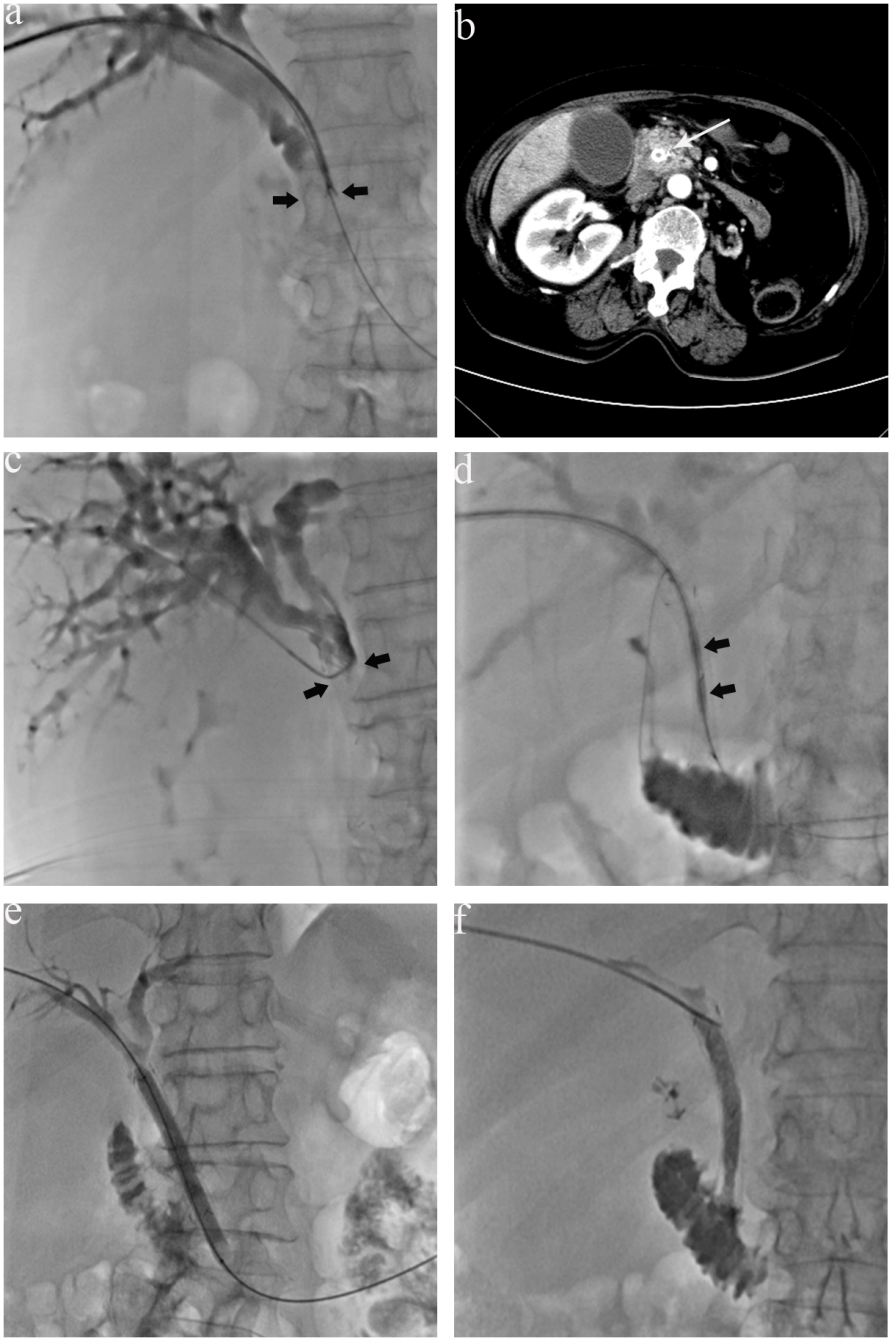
A 73-year-old woman with a highly differentiated adenocarcinoma of the middle third of the bile duct was underwent PTCB for pathogic diagnosis, at the site of biliary obstruction due to the tumor (arrows) (a). (b). Four months after biliary stent implantation, a contrast-enhanced CT showed dilated intrahepatic biliary ducts, a narrowing of the diameter of stent at the middle bile duct, and enlargement of the size of the tumor with obvious enhancement (long arrow). (c) Preablation PTC revealed stent blockages (arrows). (d) Intraductal RFA was performed with a percutaneous RFA catheter using a 0.035 inch guide wire. The two electrodes (arrows) of the RFA catheter were positioned in the area of the occlusion. (e) A balloon catheter moved back and forth through the stent into the duodenum to remove ablated tissue and debris from the stent. (f) Post-ablation PTC revealed the obstruction has been relieved.
